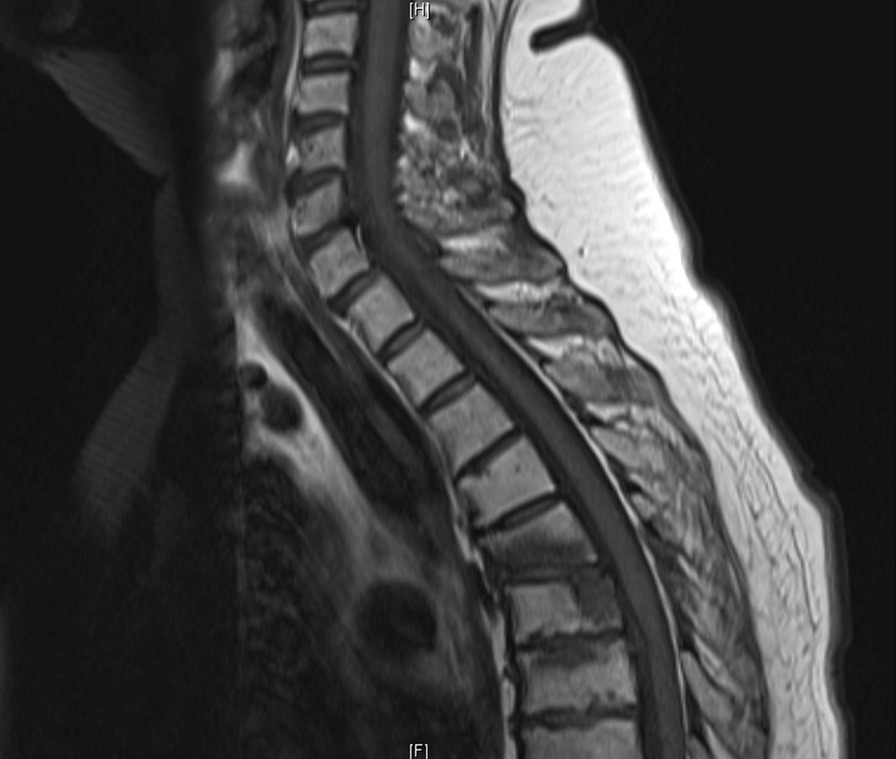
T2 (right) MRI sequence at 3 months showing excellent response to phase II boost treatment in Patient A
